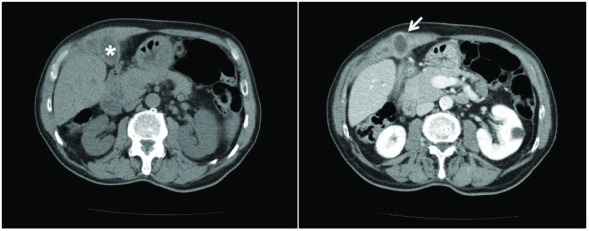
Abdominal computed tomography images. Left image, * indicates the gallbladder; right image, the arrows indicates the fistula.
